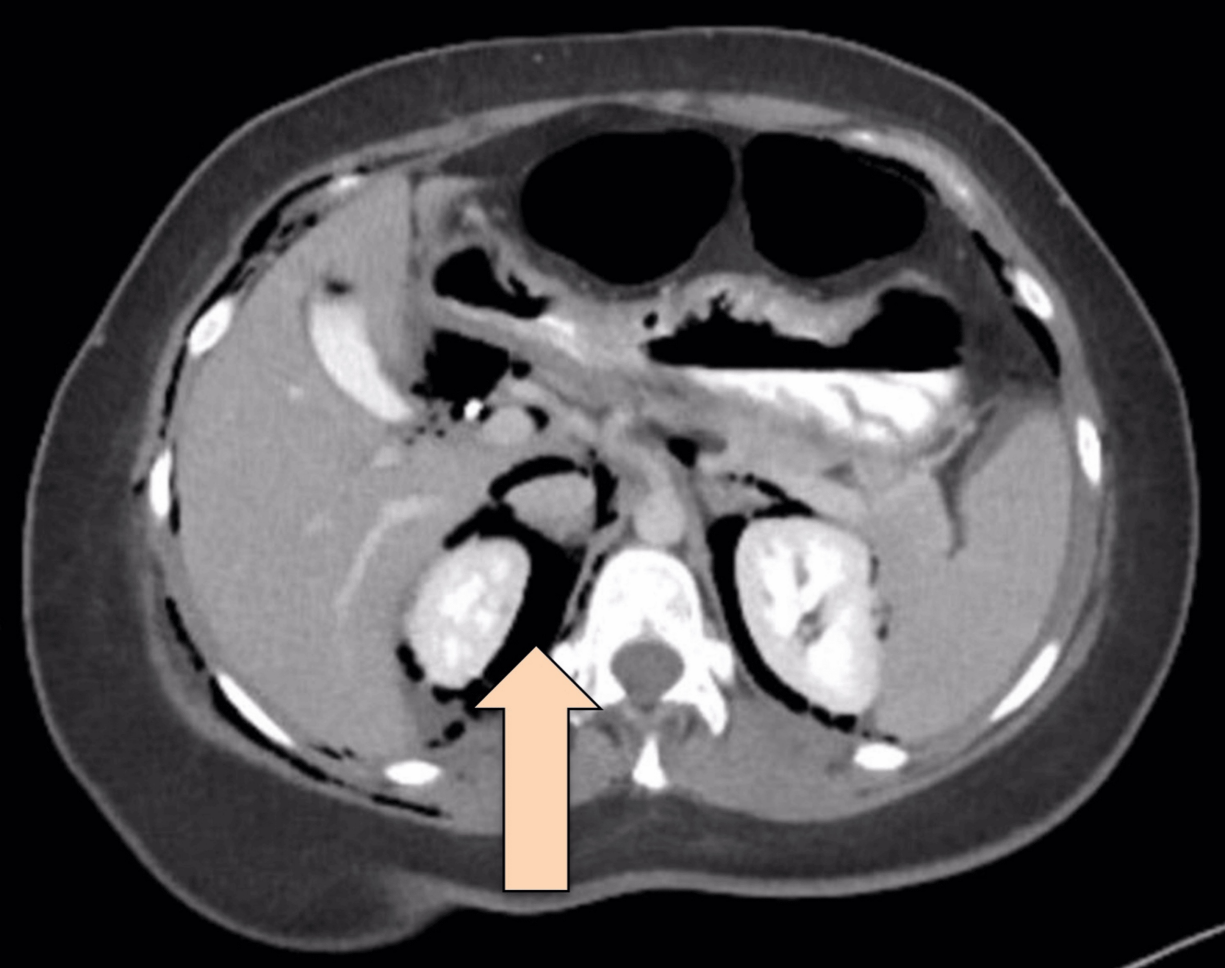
Axial view of CT abdomen with IV and oral contrast post-ERCP showing extensive pneumoperitoneum The arrow is pointing at the extensive pneumoperitoneum CT: computed tomography; IV: intravenous; ERCP: endoscopic retrograde cholangiopancreatography
